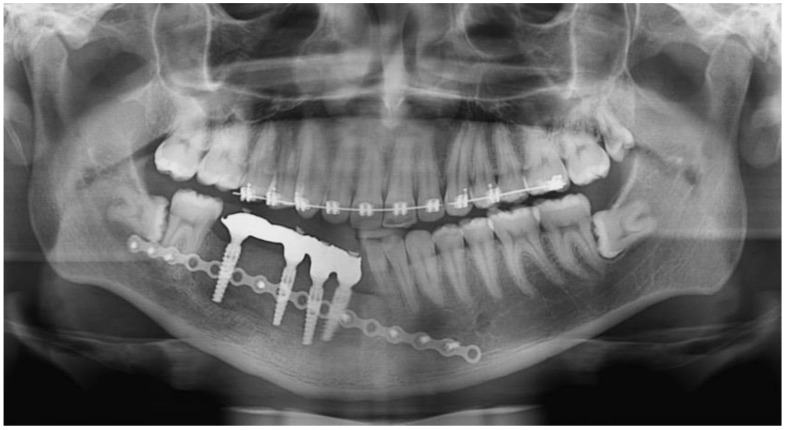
Panoramic radiographic view.
